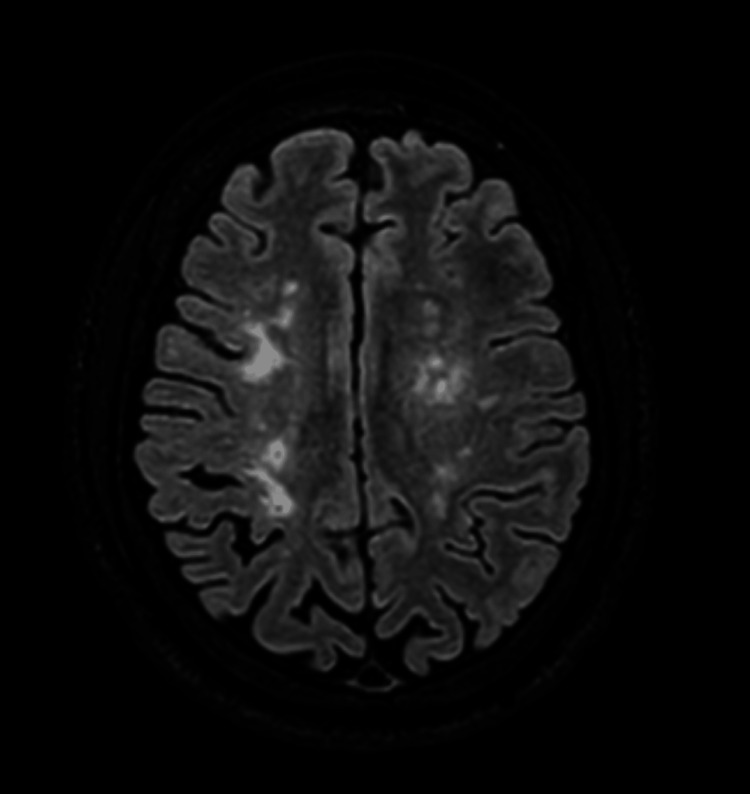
Axial FLAIR image demonstrates parenchymal atrophic changes disproportionate to the patient’s age, along with multiple hyperintense foci consistent with chronic small vessel disease and underlying vascular risk factors
